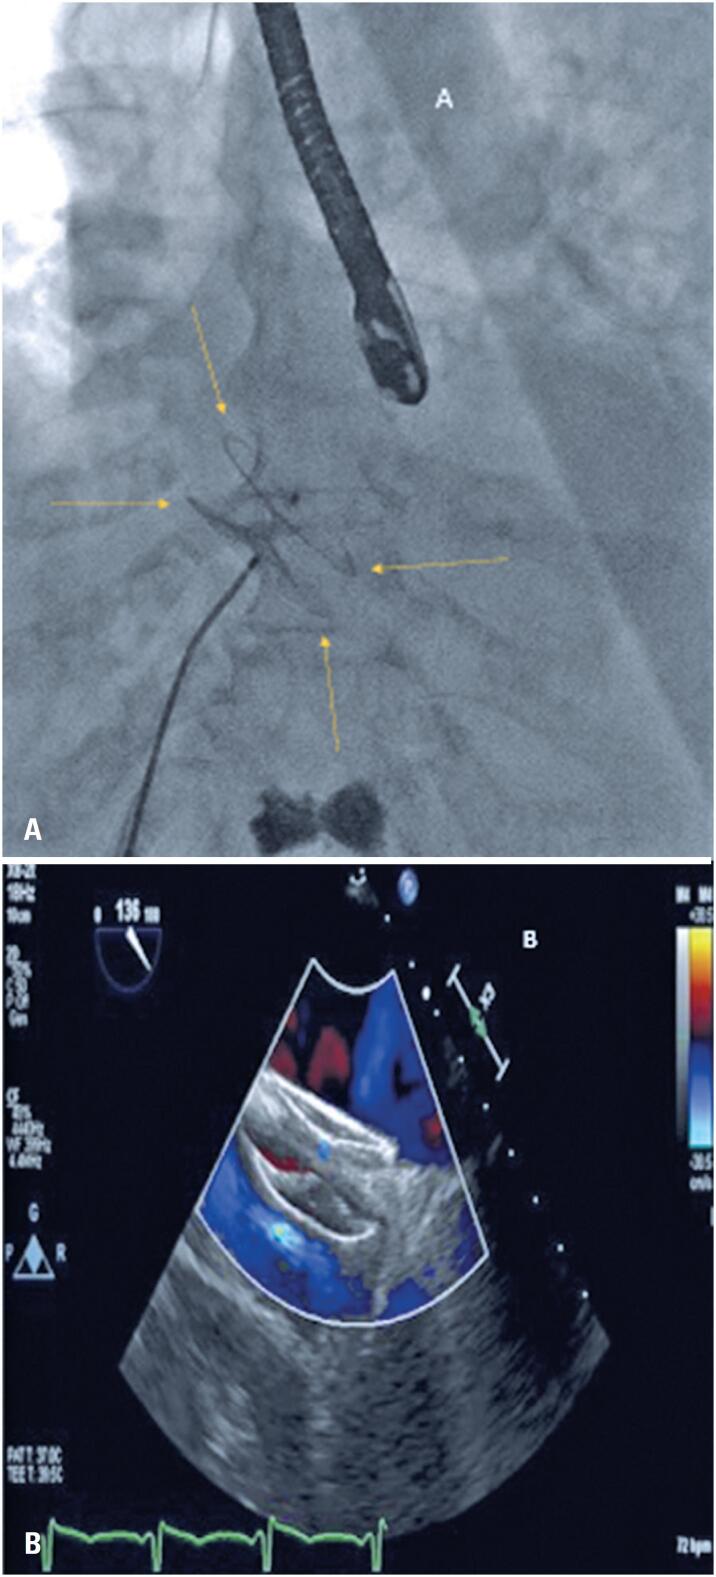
A) Fluoroscopy showing a 40-mm Amplatzer PFO device; B) Transesophageal echocardiogram with color Doppler showing minimal residual shunting after the device was deployed
